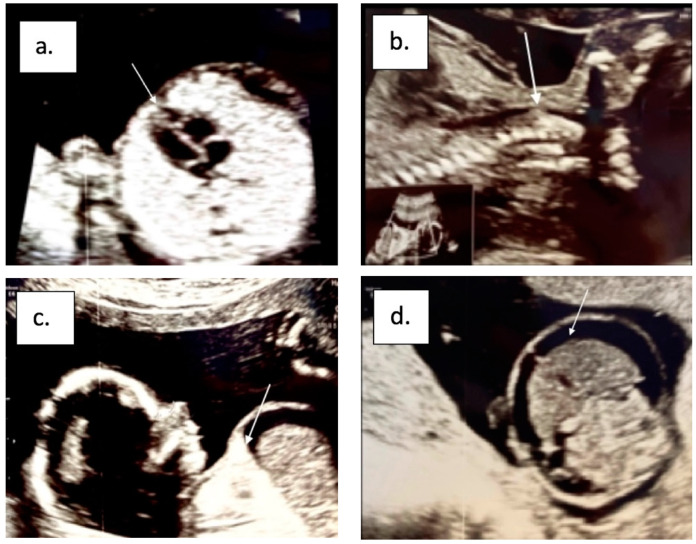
Antenatal sonography showing (a) large hyperechoic lungs and squeezed heart, (b) tracheal obstruction, (c) flattened diaphragm, and (d) ascites.
